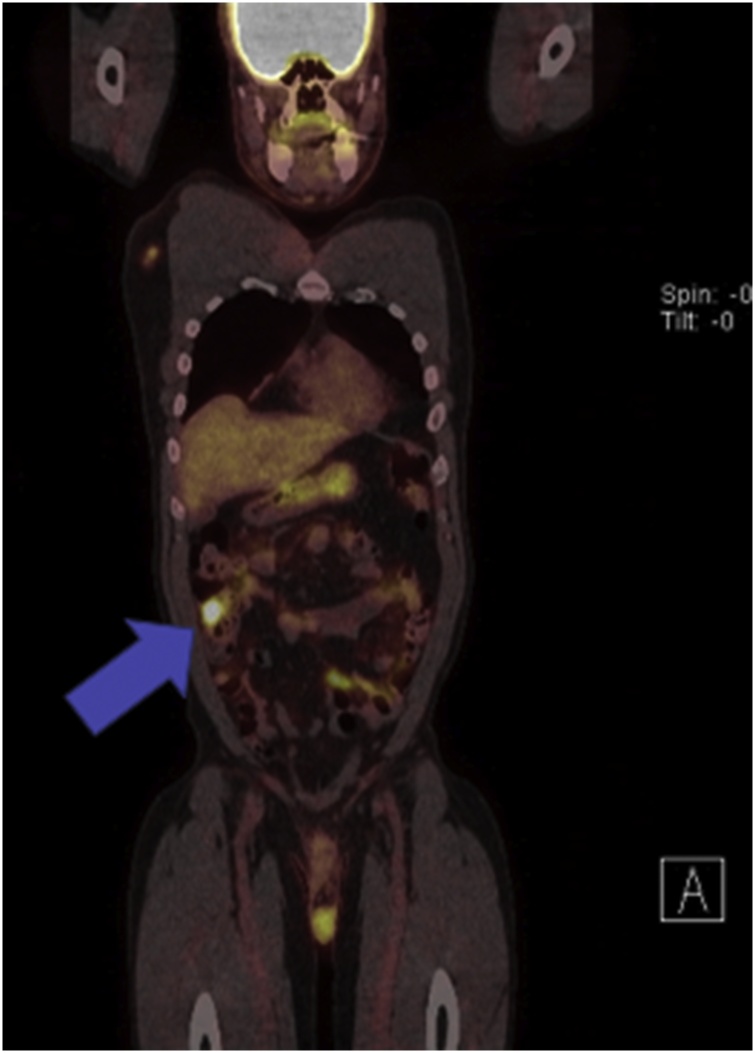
PET-CT scan of chest, abdomen, and pelvis. Coronal reformatted image obtained 90 min after IV administration of 14.0 mCi of F18-FDG demonstrating a solitary focus of intense activity noted within the cecum with a maximum standardized uptake value of 9.6 (denoted by the blue arrow). At the time, this was suspicious for a colonic primary tumor.
